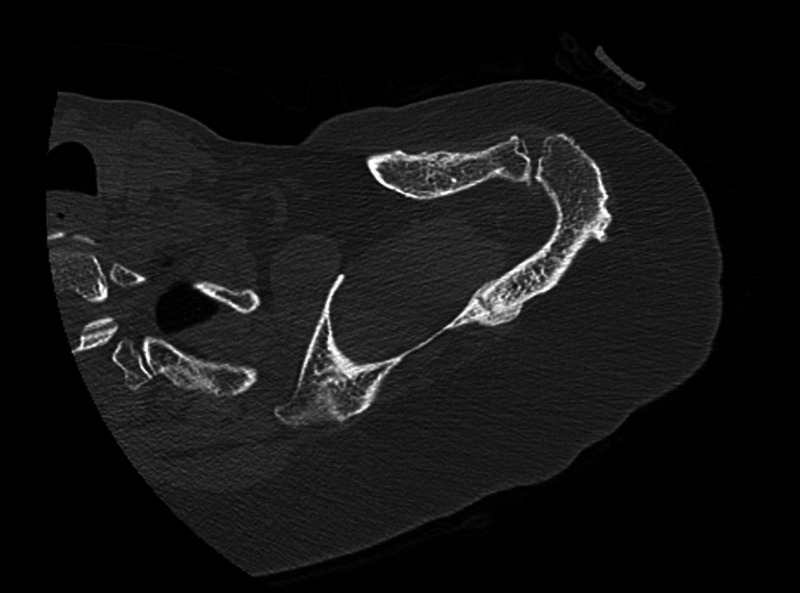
Axial section demonstrating a healed scapular spine fracture following EXOGENÒ therapy EXOGENÒ Ultrasound Bone Healing System, Bioventus, Durham NC, Netherlands
